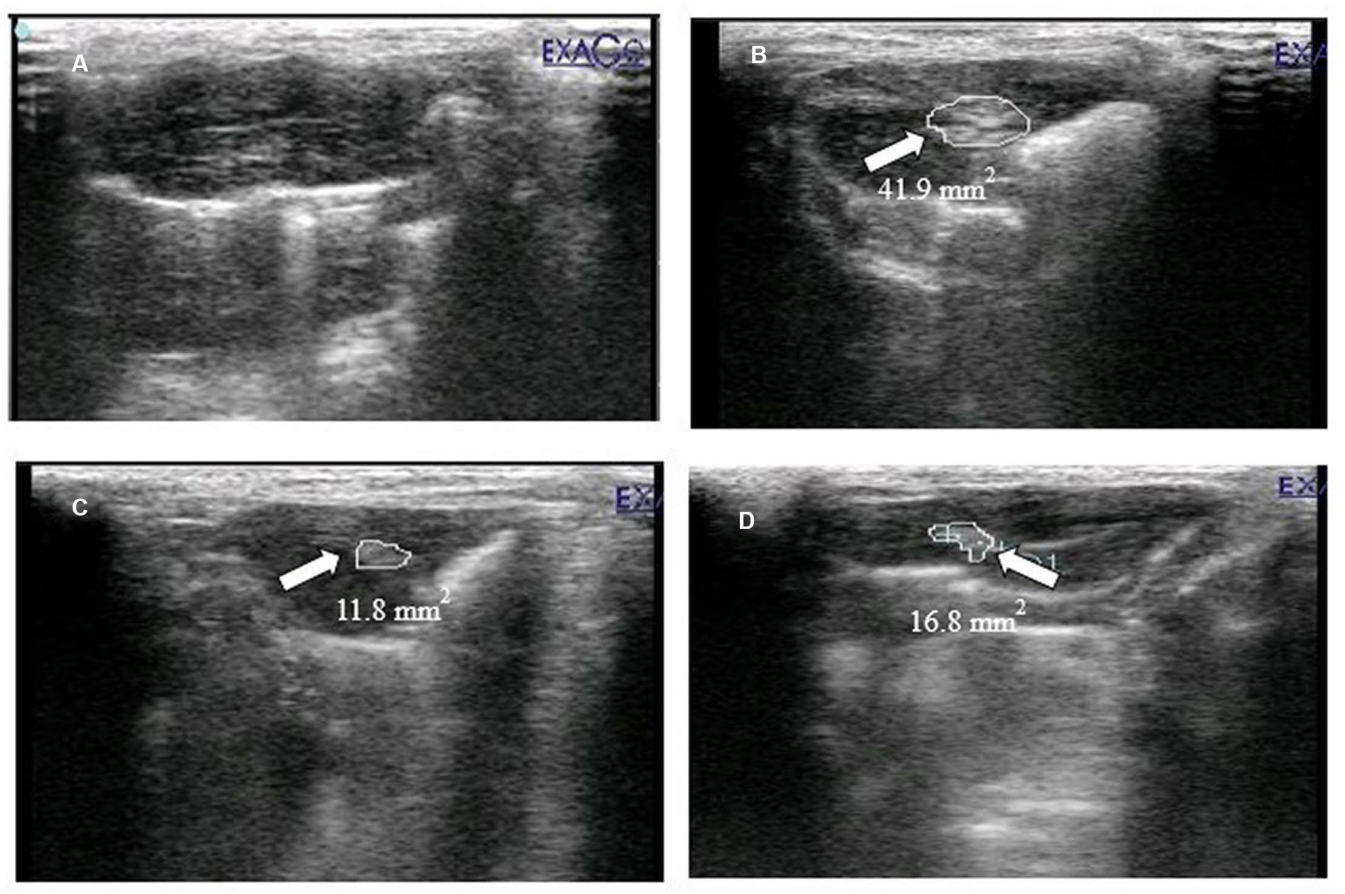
Ultrasound imaging of (A) normal control (HCGo), (B) negative control (HCG_1_ = DMBA), (C) standard group (HCG_2_ = DMBA+Doxorubicin) and (D) treatment group (HCG_3_ = DMBA+*P. avium* extract).
